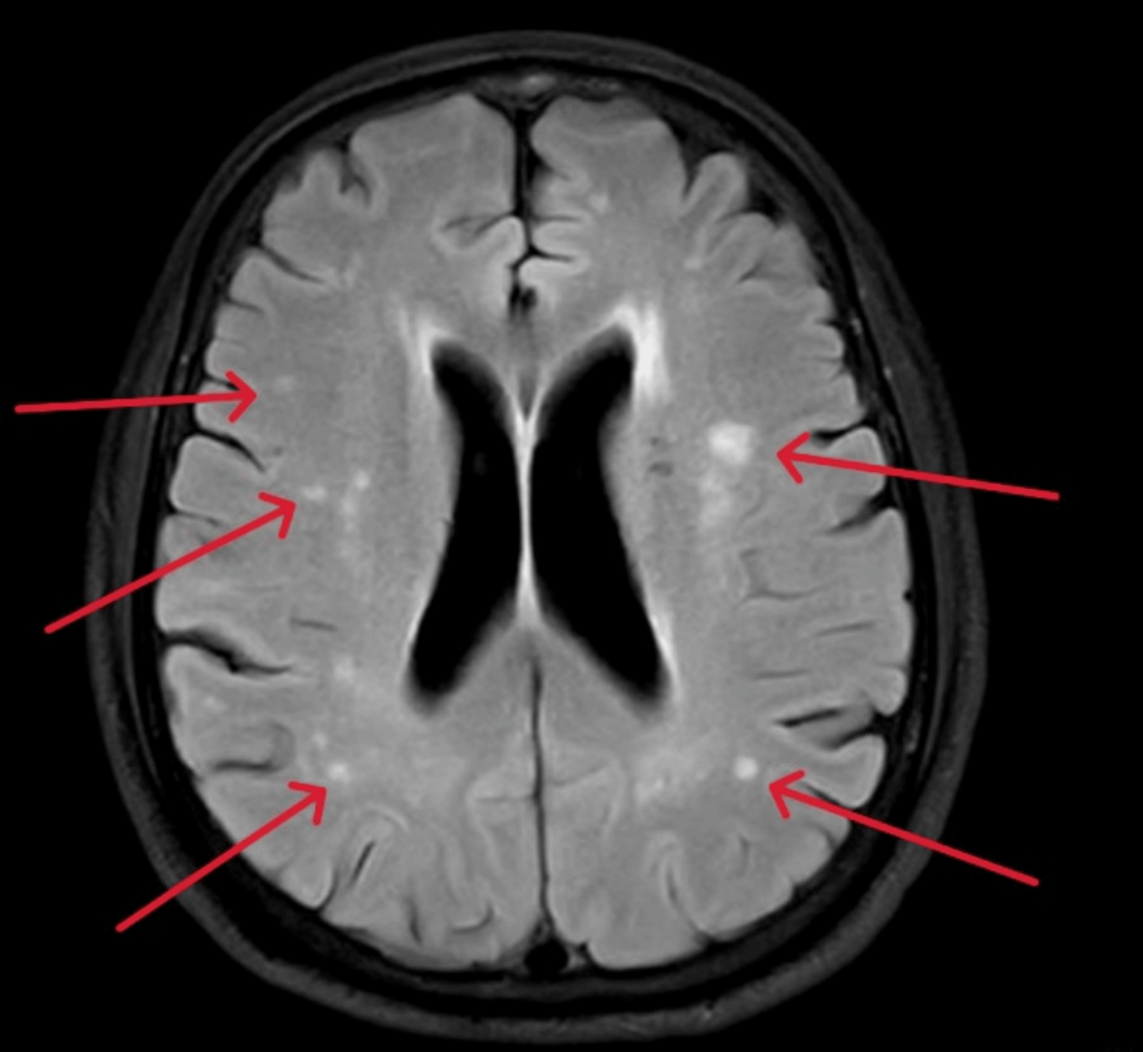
Magnetic Resonance Imaging axial view (Brain) The brain MRI revealed multifocal patchy hyperintense areas and foci, consistent with a recent ischemic infarct (red arrows)
